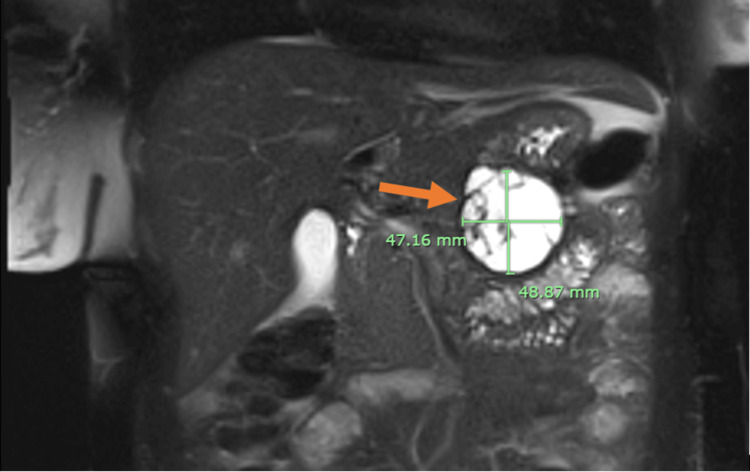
MRI image Arrow indicating a well-defined, rounded multicystic lesion in the pancreatic tail, measuring approximately 48 x 49 mm
